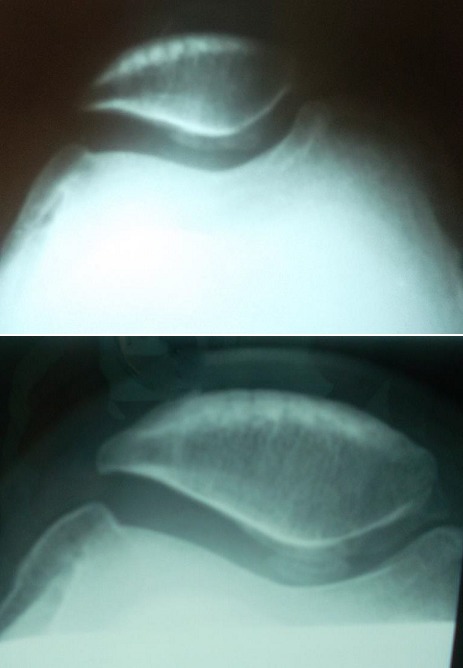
Radiographies fémoro-patellaires à 30° de flexion du genou droit, en pré-opératoire et au dernier recul chez un patient de notre série ayant une arthrose fémoro-patellaire associée
